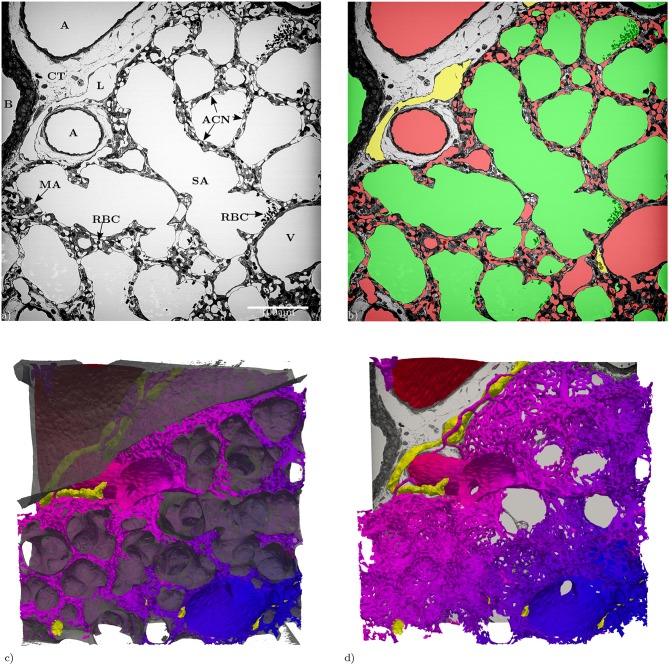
(a) Slices of the entire tissue block (525 × 525 μm) in the z-direction: A, artery; ACN, alveolar-capillary network; B, bronchiolus; CT, connective tissue; RBC, red blood cells; L, lymphatic vessel; MA, microatelectasis; SA, saccular airspace; V, vein. (b) Segmentation of the structures of interest (same slice as in a): red = blood vessels, green = airspace, yellow = lymphatic vessels. (c) 3D Surface rendering (525 × 525 × 420 μm) of the segmented structures of interest, air: semi-transparent gray, vasculature: red to blue, lymphatic vessels: yellow. (d) Like (c) without the air surface but with the slice from (a).
